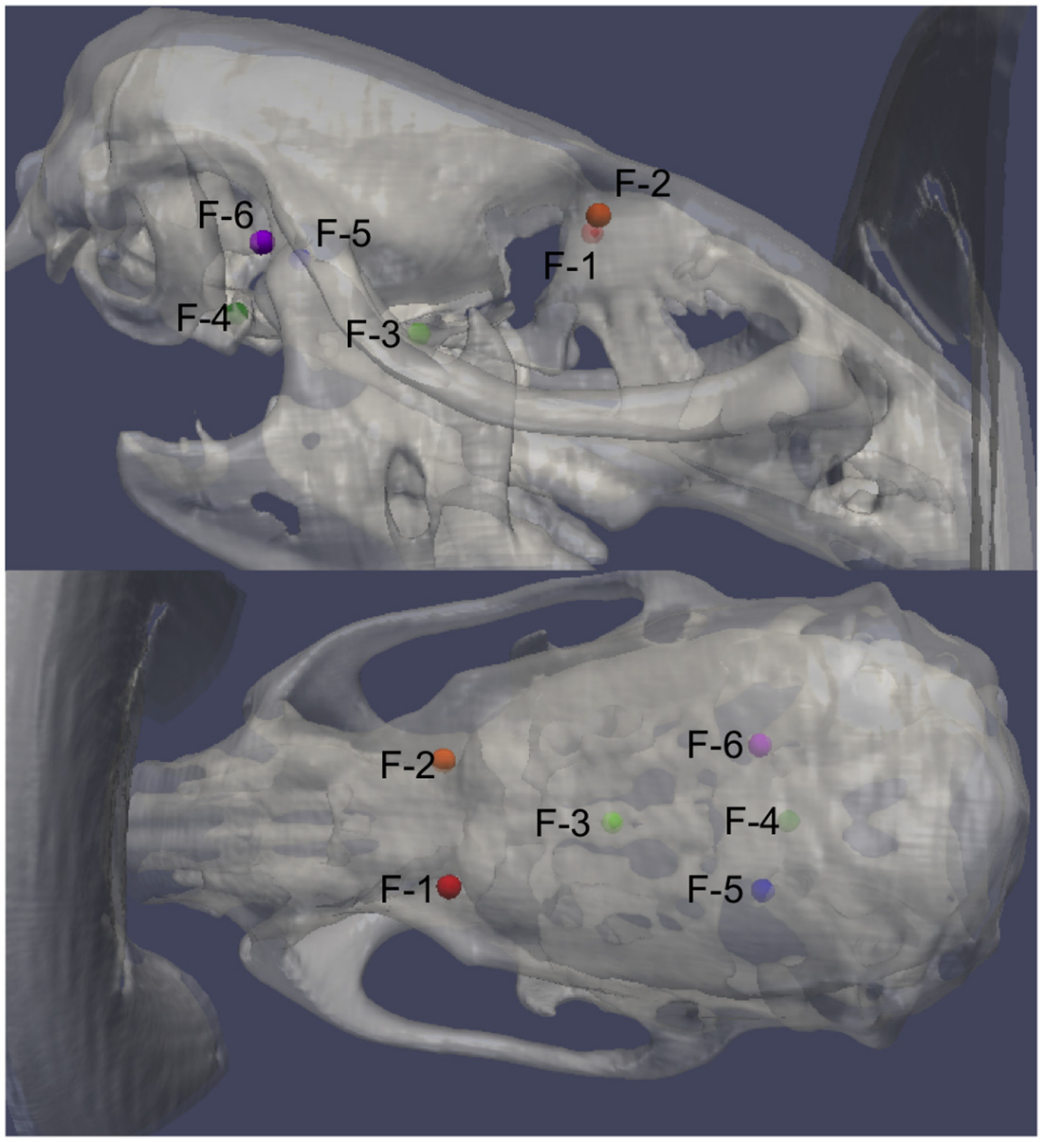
3D view of the six bony landmarks F-1 through F-6 used for registration. Please see S1 Fig and S1 Video for complete CT and MRI details of registration points.
